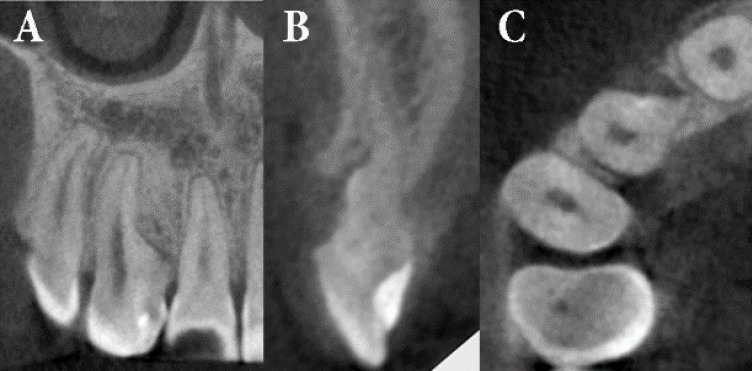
A)* No bone defect within the operation area in coronal CBCT; *B)* On the sagittal image, the trabecular bone over the root surface is apparent, the operation area seems to be filled with gingiva since removed root piece was located at the buccal area. Clinical examination of the related area revealed no pathological periodontal pocket; *C)* The overhanging tooth structure was elongated mesially towards the distal in the axial view
